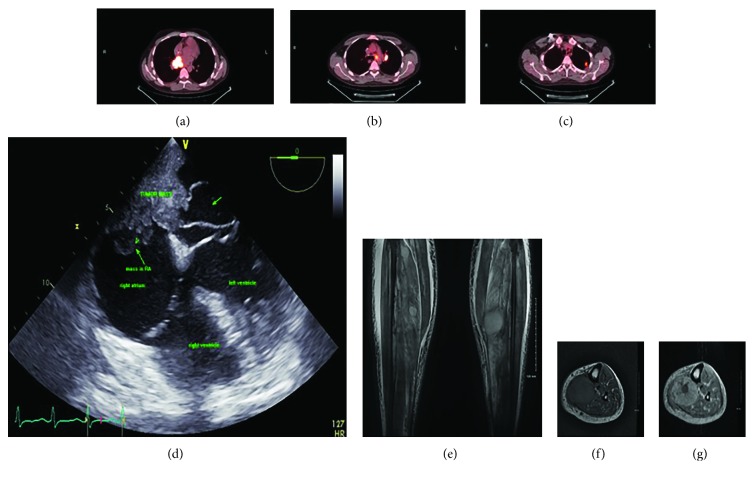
(a–c) PET scan images demonstrate increased uptake within nodes in the aortic pulmonary window, left hilum, and a mass in the left upper lung. (d) Transesophageal echocardiography shows large cardiac mass invaded to the intra-atrial septum and right and left atrium with cystic lesion protruding to the left atrium. (e–g) MRI with and without contrast shows multiple enhancing neoplastic masses in the muscle compartment in both lower extremities which are believed to be due to metastatic lesions.
